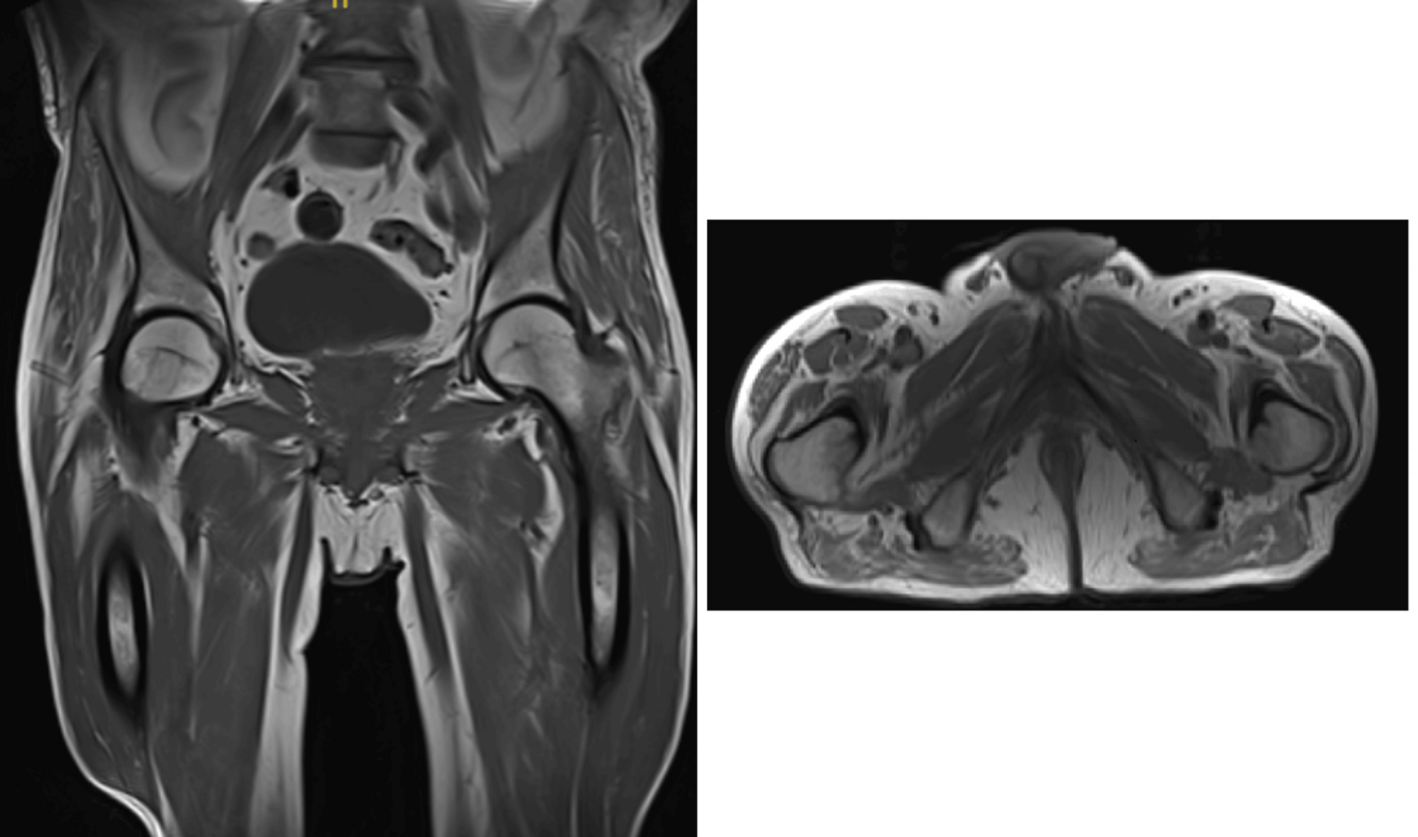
Magnetic resonance imaging (MRI) of the lower limbs showing diffuse polymyositis involving the pelvic and both leg muscles.
